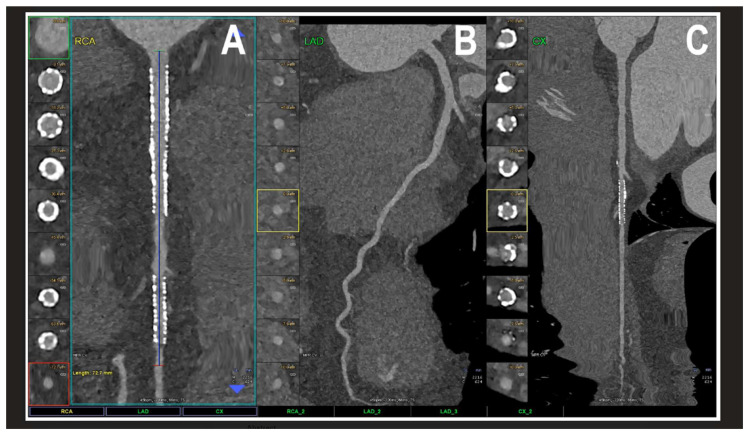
A cardiac PCCT visualization of the coronary stents and stented lumen. There are two stents at the level of the proximal and middle RCA (A) and one stent on the marginal branch of the left LCx (C); the LAD (B) is normal without any detectable atherosclerotic disease. All stents are perfectly visualized in their inner struts and also in their inner lumen, which is difficult to visualize with standard cardiac CT. PCCT—photon counting CT, LAD—left anterior descending, LCx—left circumflex, and RCA—right coronary artery. Reprinted with permission under open access guidelines from Cademartiri et al. [15].
